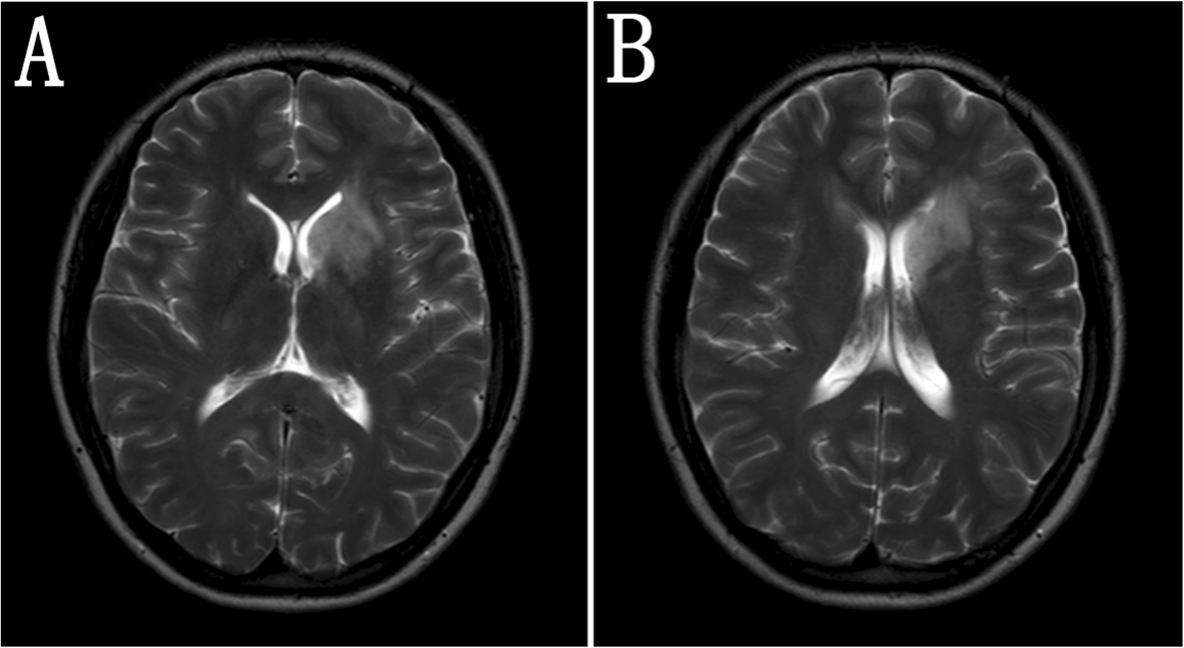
Brain MRI after treatments. Forty days after the initiation of treatments, brain MRI showed that the lesion in right frontal lobe nearly disappeared, and the lesions in left frontal lobe and left basal ganglia and callosum were reduced (a, b)
